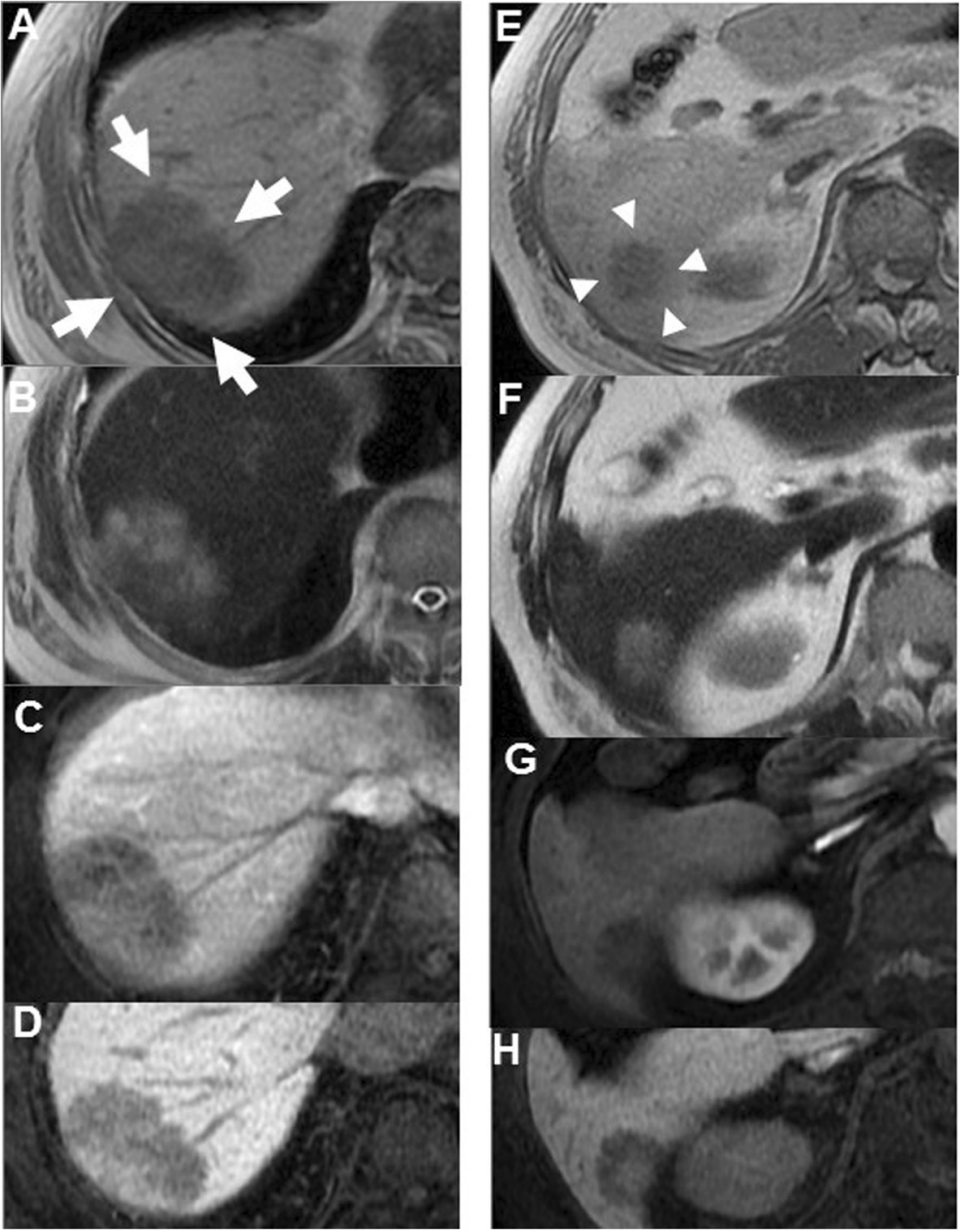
Abdominal contrast-enhanced magnetic resonance imaging (a–d: segment 7, e–h: segment 6). a (white arrows), e (white arrowheads) T1-weighted image. b, f T2-weighted image. c, g Arterial phases on T1-weighted image. d, h Parenchymal phases on T1-weighted image. a, b, e, and f Both liver tumors show the same intensity patterns on T1-weighted image and T2-weighted image. c, g However, in the arterial phase on T1-weighted image, the tumor in segment 7 shows heterointensity, but the tumor in segment 6 shows central enhancement. d, h In the parenchymal phase of T1-weighted image, the tumor in segment 7 shows more heterointensity, and the tumor in segment 6 shows more central enhancement
